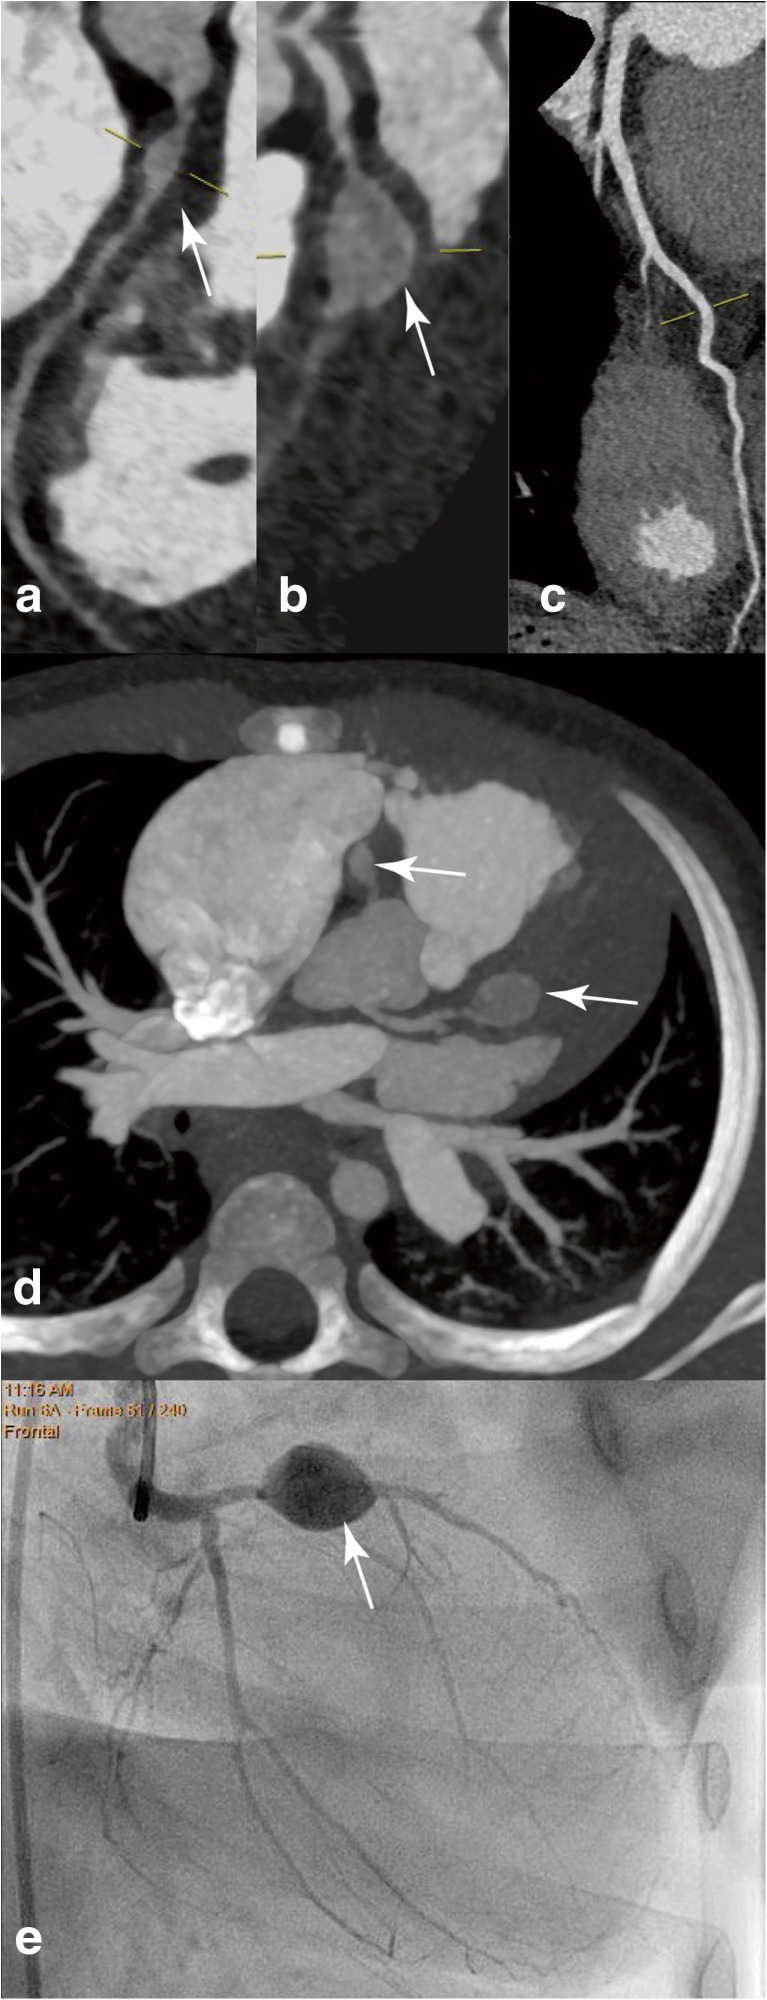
Imaging techniques used for Kawasaki disease. a, b, d, e display coronaries of the same patient with different imaging techniques. a Curved multi-planar reformat of the coronary computed tomography angiography (cCTA) scan shows an aneurysm of the right coronary artery. b A giant aneurysm of the left anterior descending artery. c A normal left anterior descending artery. d Thin slab maximum intensity projection of the aneurysmatic proximal right coronary artery and left anterior descending artery. e A clearly depicted giant aneurysm of the left anterior descending artery, visualized with coronary angiography
